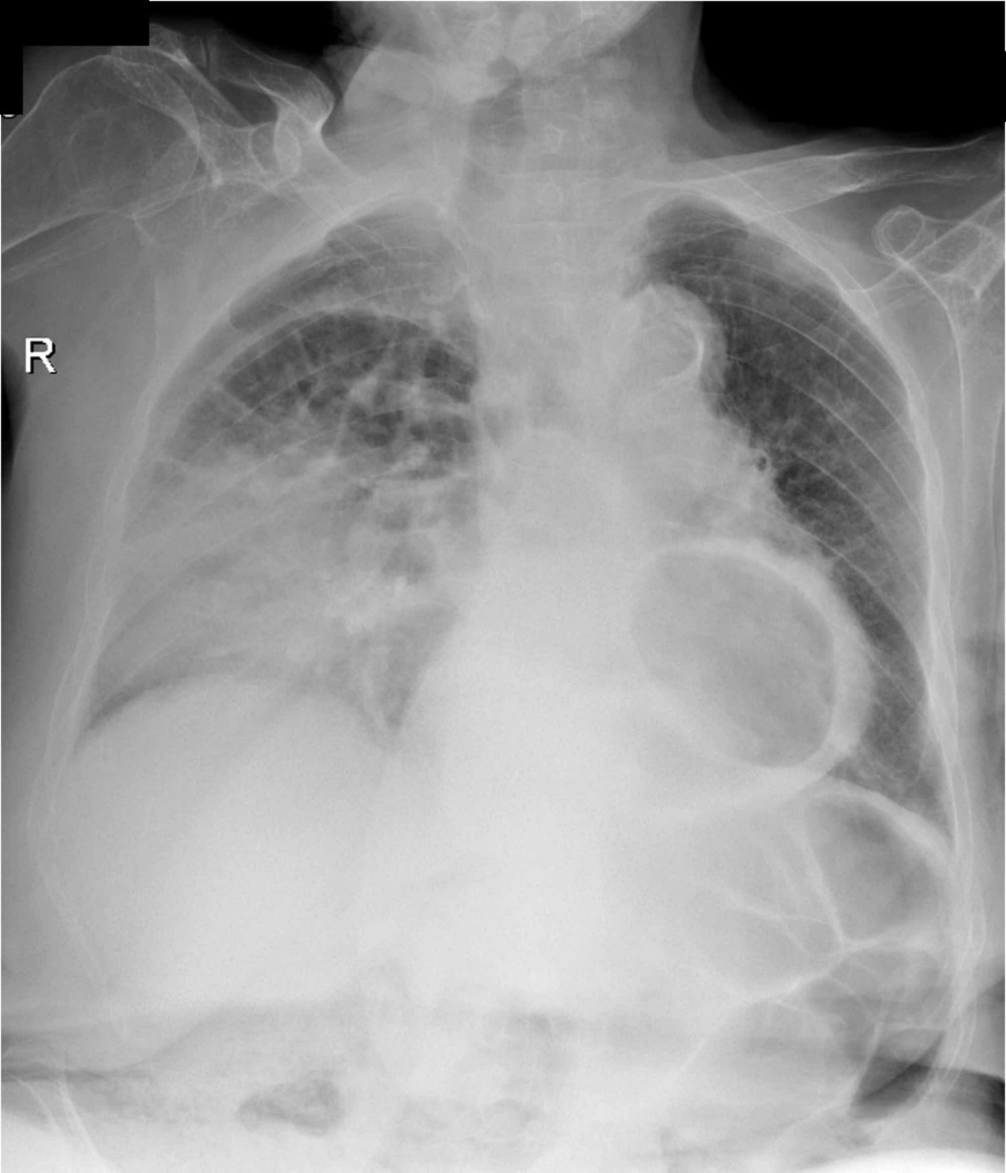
Chest radiograph suggesting the presence of the small bowel inside the thoracic cavity, collapsing the right lung
